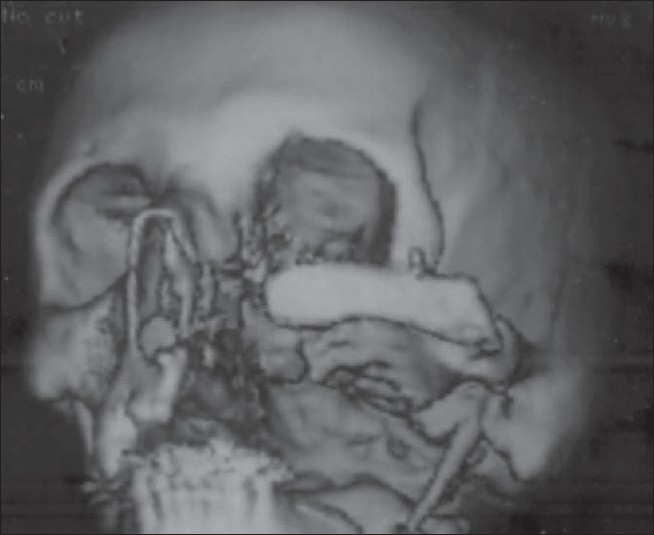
Computed tomography scan of the same patient 6 months postoperatively showing well-incorporated bone
